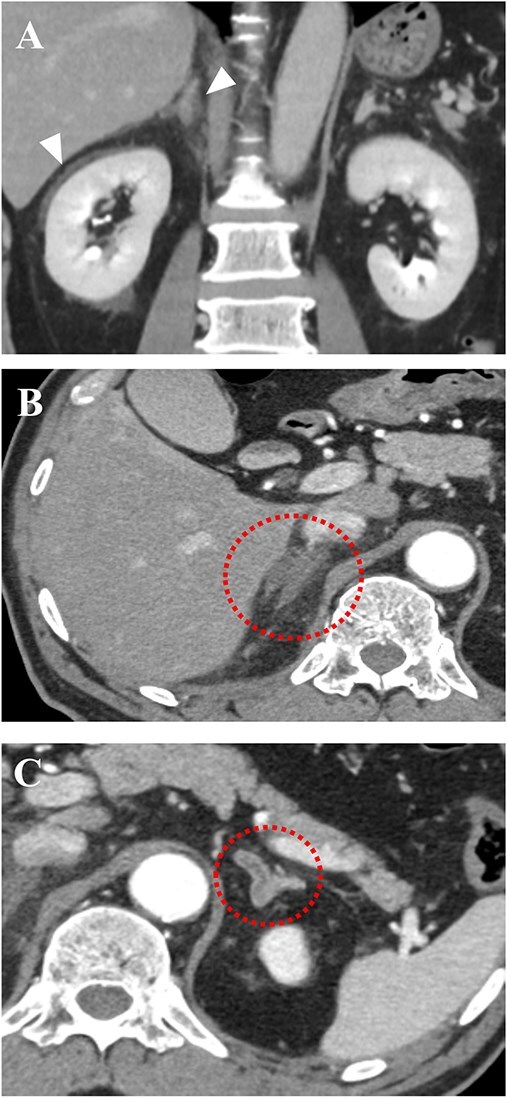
Abdominal contrast-enhanced computed tomography (CT) scans on admission. (A) Sagittal section image. Arrows indicate panniculitis around the right kidney and adrenal gland. (B and C) axial section images. Bilateral enlarged adrenal glands with no enhancement after contrast injection. On the left adrenal gland, CT scan shows no parenchymal enhancement with minimal peripheral capsular enhancement (‘capsular sign’).
